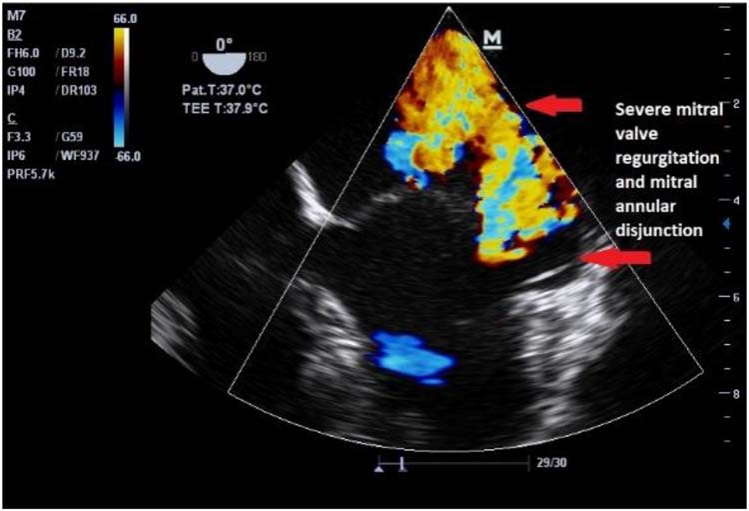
Preoperative echocardiography of patient 2.
